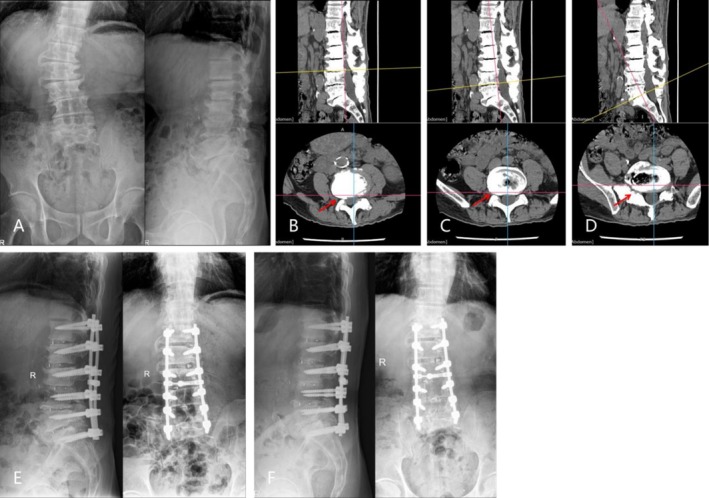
(A) Preoperative coronal and sagittal lumbar radiographs of a patient showing degenerative scoliosis. (B–D) Preoperative CT revealed multiple stenoses of intervertebral disk spaces and intervertebral foramina, most prominently manifested on the right side at the L3/4, L4/5, and L5/S1 levels. (E) The patient was treated with long‐segment PLIF; postoperative sagittal X‐ray revealed restoration of intervertebral disk height, with correction of the coronal Cobb angle. (F) Postoperative radiographs of the same patient taken 1 year postoperatively showing the correction of the Cobb angle and adjacent segment degeneration at the top.
